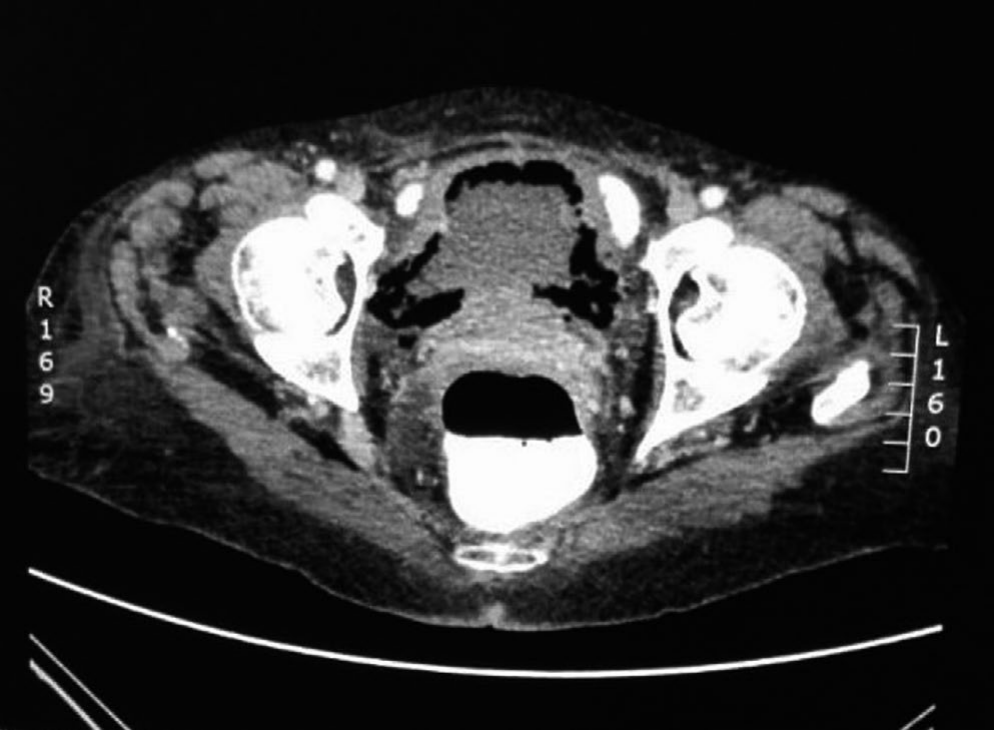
Axial view of CT abdomen/pelvis demonstrating the presence of extensive air in the bladder wall
